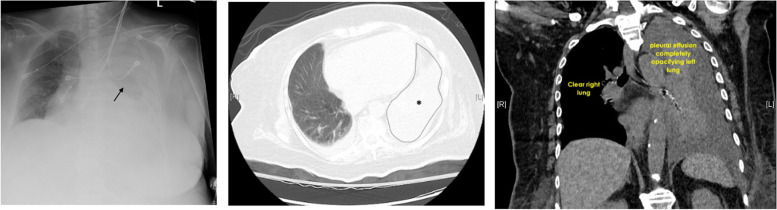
AP chest radiograph, and axial and saggital views of chest CT demonstrating left lung white out
